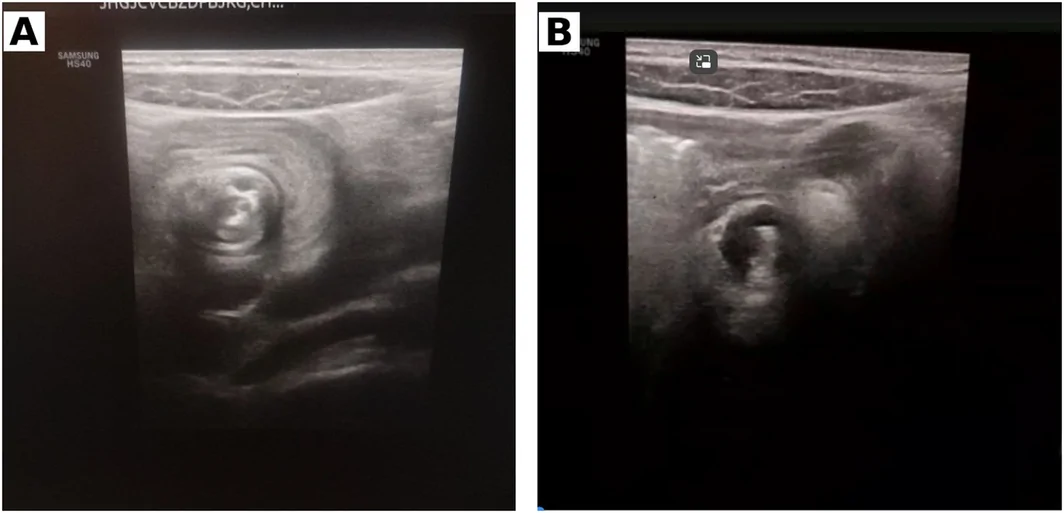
Transverse abdominal ultrasound images demonstrating the whirlpool sign. (A) Clockwise twisting of the superior mesenteric vein (SMV, arrow) around the superior mesenteric artery. (B) The SMV (arrow) and surrounding mesentery forming the characteristic swirling configuration around the SMA axis.
